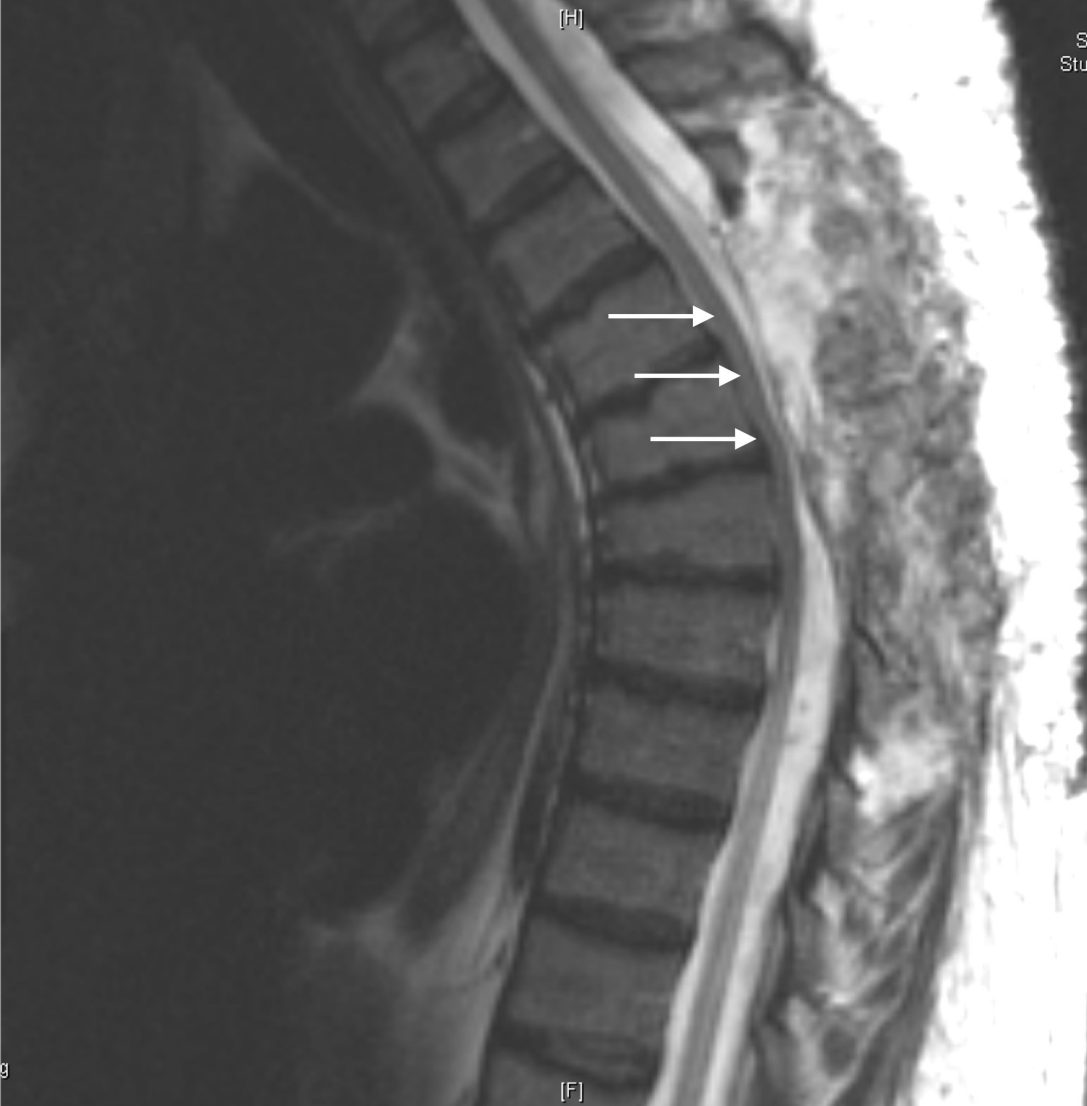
Post-Operative Sagittal MRI of Thoracic Spine
